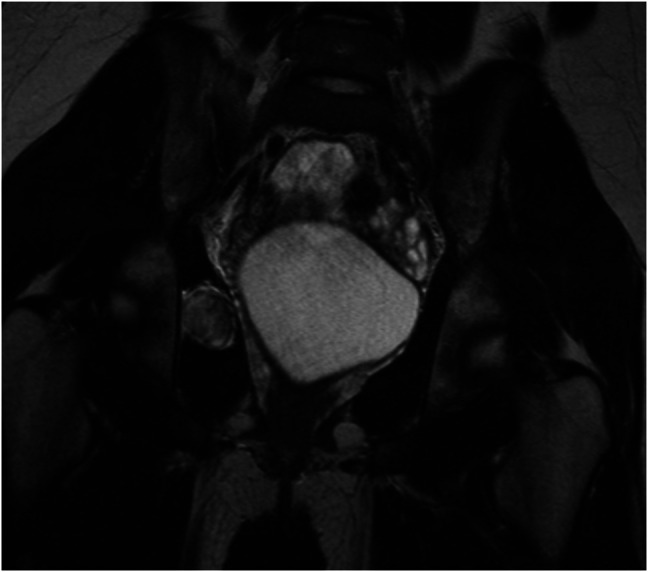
Coronal MRI view showing the right obturator internus intramuscular myxoma.
